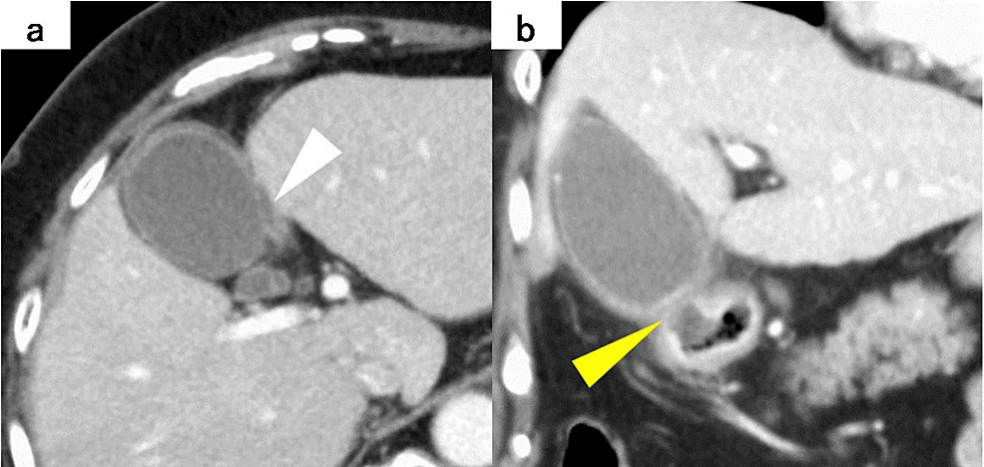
Contrast-enhanced CT Axial (a): Abdominal CT showed a thickened gallbladder wall and a small amount of pericholecystic fluid (white triangle). Coronal (b): There was a partial mucosal defect on the first portion of the anterior duodenum, suggestive of a duodenal ulcer (yellow triangle)
